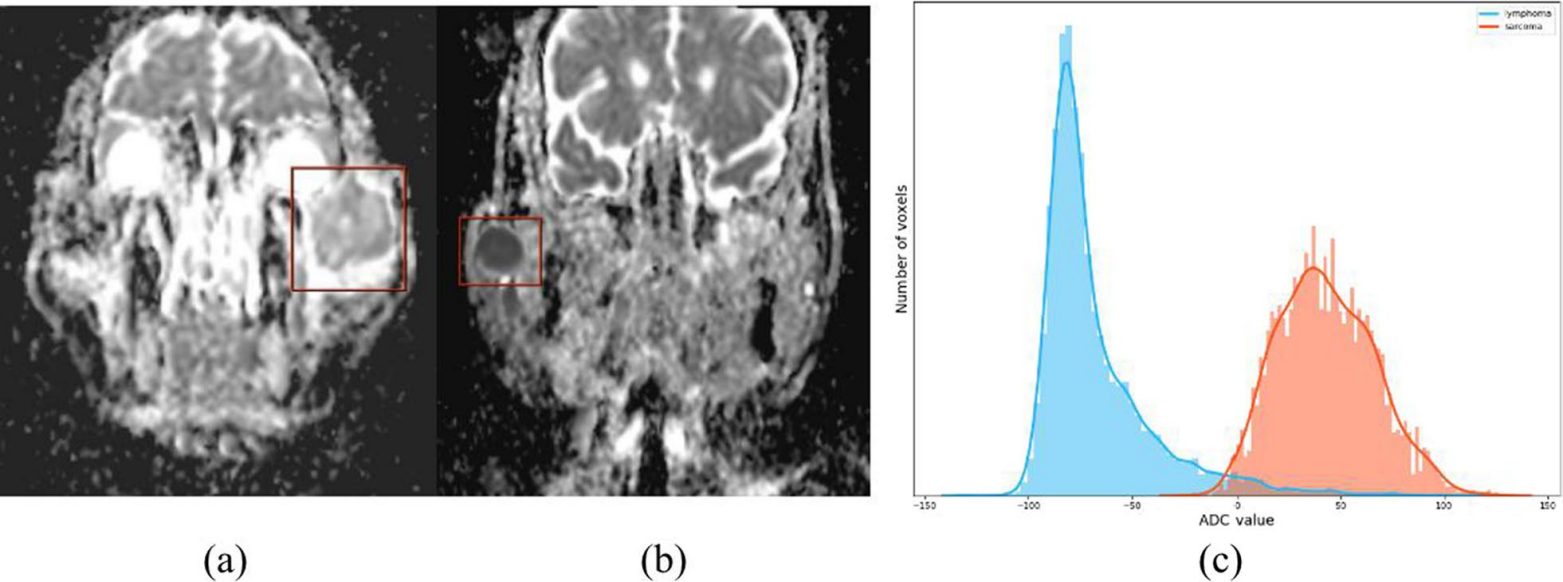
DWI-ADC images of a scarcoma and b lymphoma. a A 28 years old female patient with a sarcoma within the left zygoma, DWI-ADC image the lesion appears relatively inhomogeneous slightly higher signal compared to the adjacent muscle. ADC value is about 1.12 mm^2^/s; b a 67 years old male patient with a lymphoma within the right parotid gland, DWI-ADC image the lesion appears relatively homogeneous lower signal compared to the adjacent muscle. ADC value is about 0.54 mm^2^/s; c lymphoma correlating with an ADC_mean_ in the lower range, positive skew (high skewness), and a steep curve (high kurtosis). Sarcoma correlating with a higher ADC_mean_ value, a negative skew (low skewness), and a flatter shape (low kurtosis)
